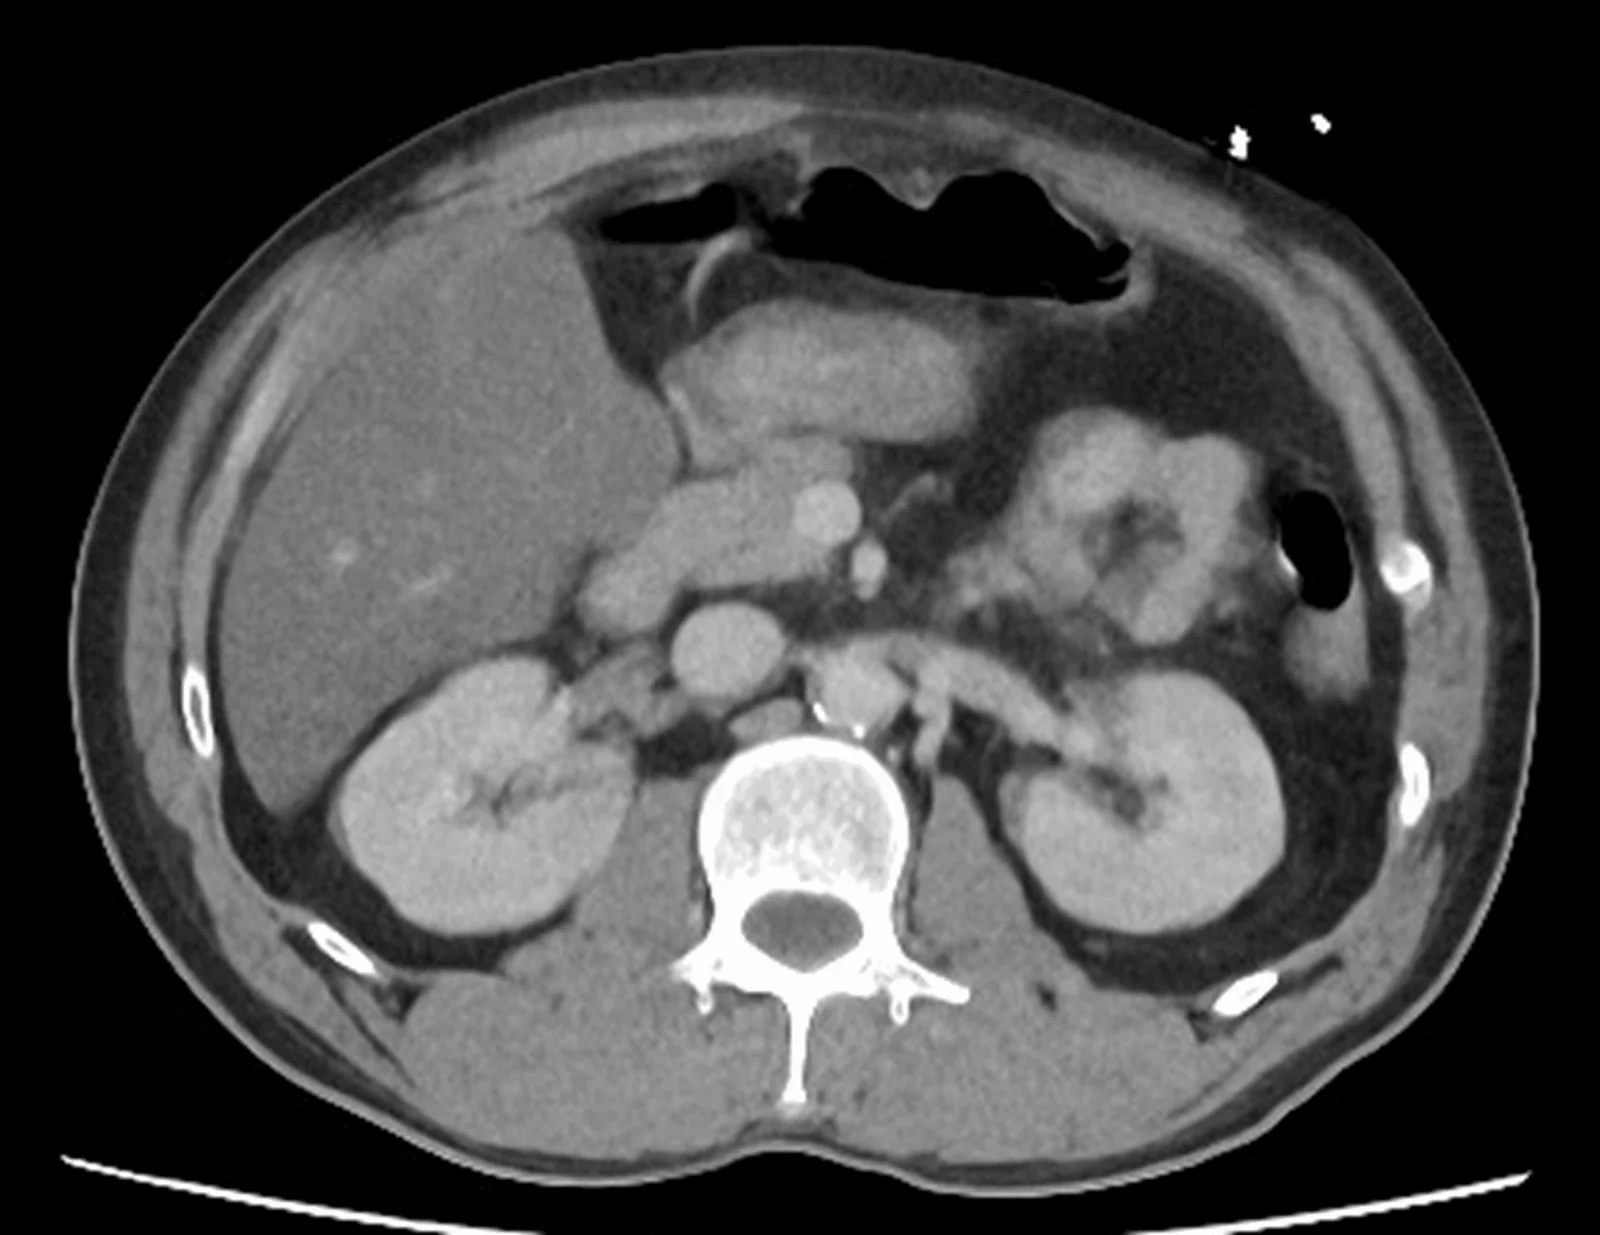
Contrast-enhanced axial CT image of the abdomen Representative contrast-enhanced axial CT image of the abdomen obtained during evaluation for intra-abdominal causes of severe lactic acidosis. No acute intra-abdominal abnormality, bowel ischaemia, or infective focus was identified on CT imaging.
